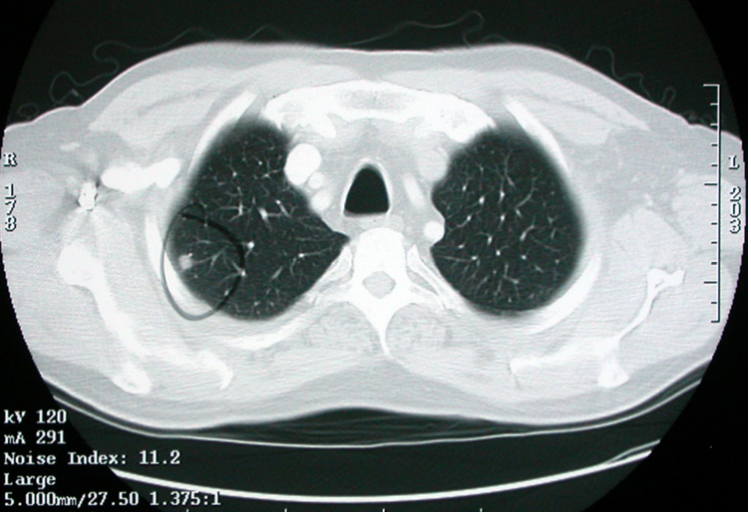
Chest CT scan showing a 1 cm right upper lobe nodule.
